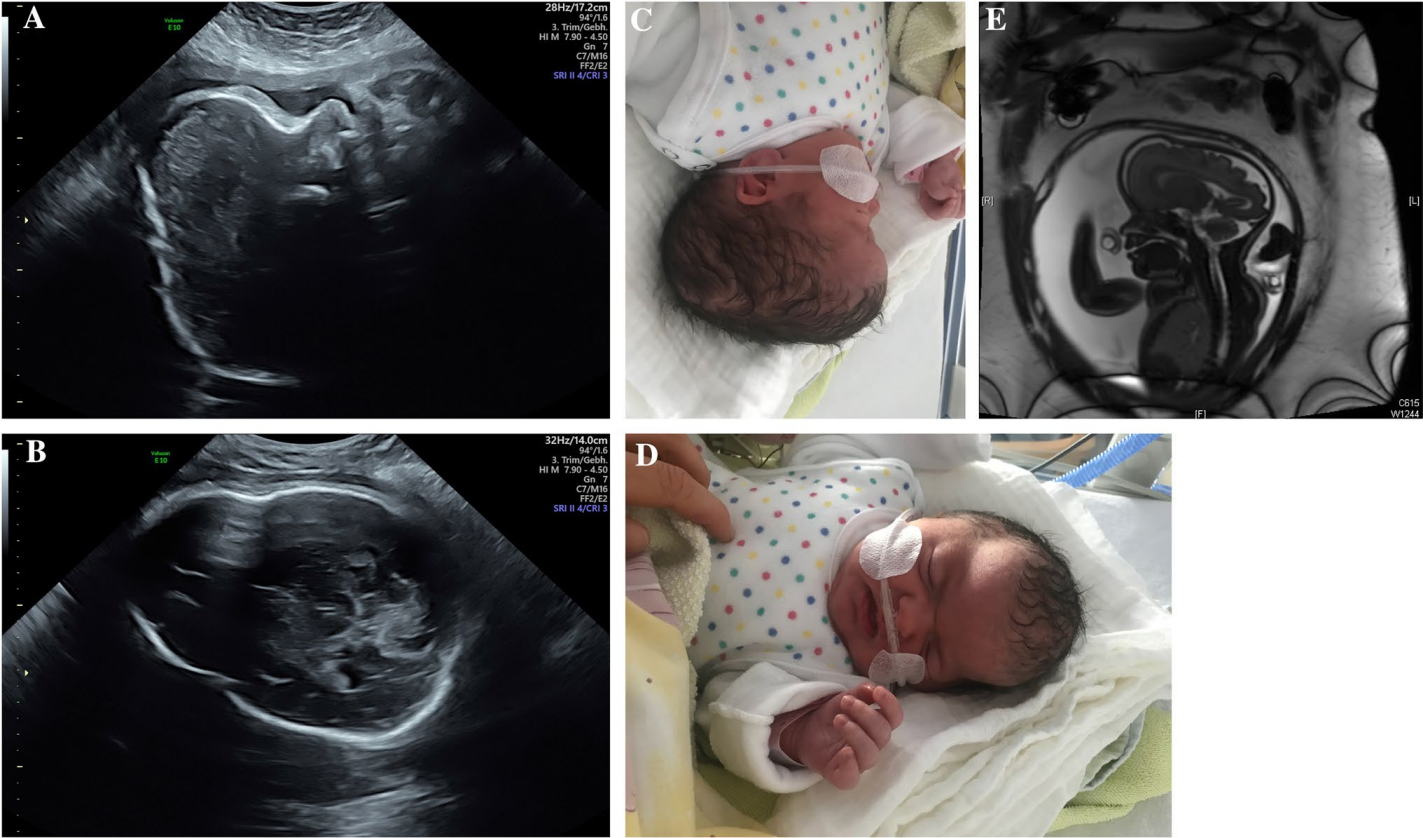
Child with suspected Apert syndrome. The prenatal ultrasound exam in 31 + 3 weeks of gestation shows a prominent shape of the skull with bicoronal and sagittal craniosynostosis as well as frontal bossing (a, c). The prenatal MRI confirms the findings (e). After the birth, a scaphocephaly with a long and narrow skull and high forehead is seen (d). The genetic examination showed a mutation in FGFR2-gene (Pro253Leu), the father had the same mutation. At this amino acid position is the pathogen mutation p.Pro253Arg located, which leads to Apert syndrome
